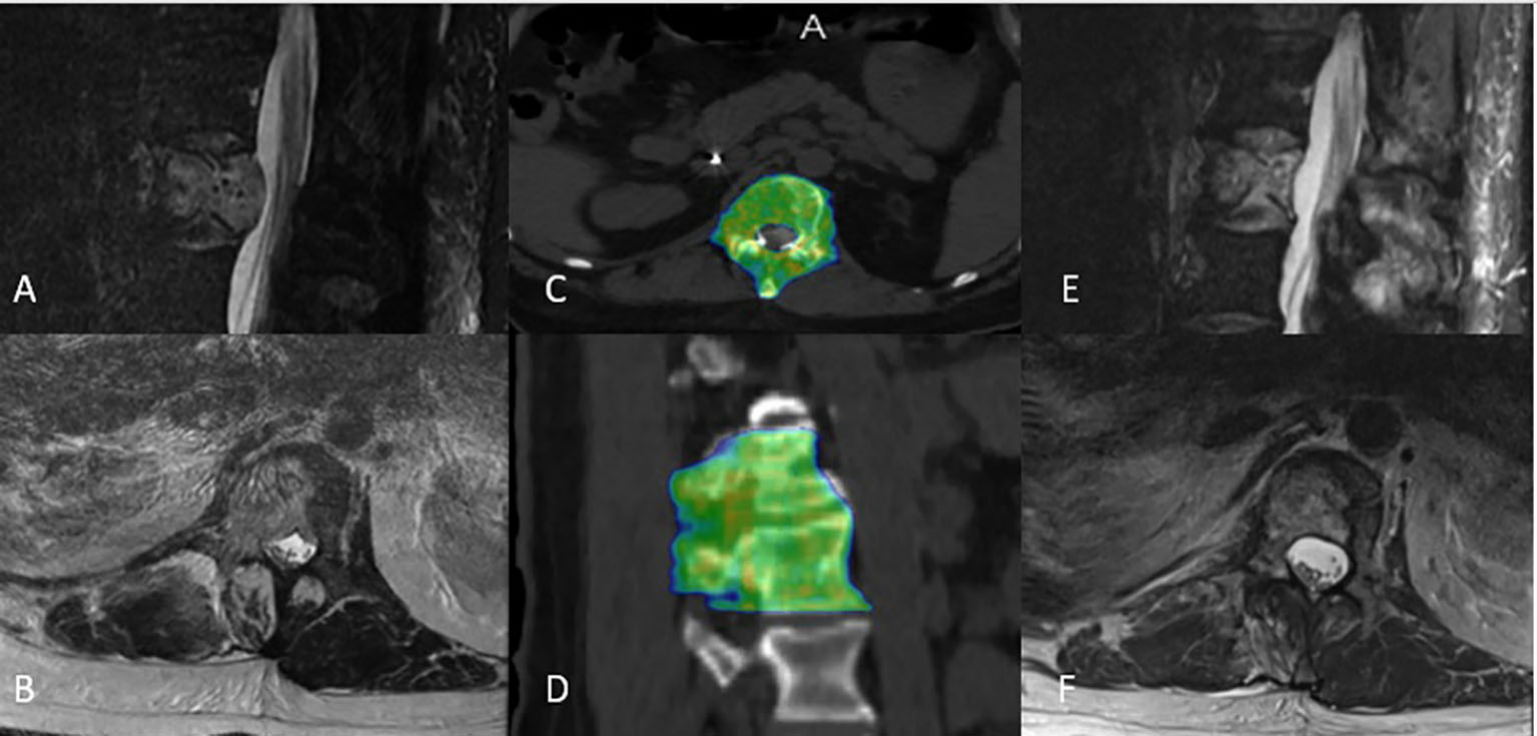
Representative circumferential stereotactic body radiation therapy plan from a patient treated to T12-L1 with (A, B) T2-weighted sagittal and axial MRI showing spinal canal narrowing due to extensive metastatic disease involving the vertebral body, right lateral compartment, and posterior compartment. (C, D) He was treated to 27 Gy in three fractions. (E, F) Surveillance T2-weighted sagittal and axial MRI showed with disease regression 4 months post-treatment.
